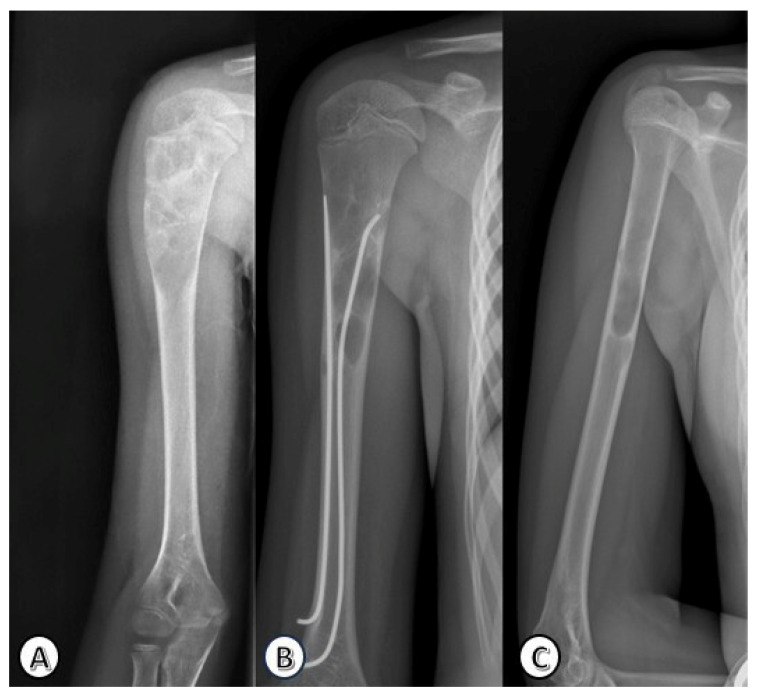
Radiographic progression of a humeral solitary bone cyst in a 15-year-old girl involving the right proximal meta-diaphysis. (A) Preoperative anteroposterior view showing the cystic lesion. (B) Radiograph after ESIN fixation. (C) Follow-up radiograph after ESIN removal, demonstrating complete cyst resolution and bone remodeling.
